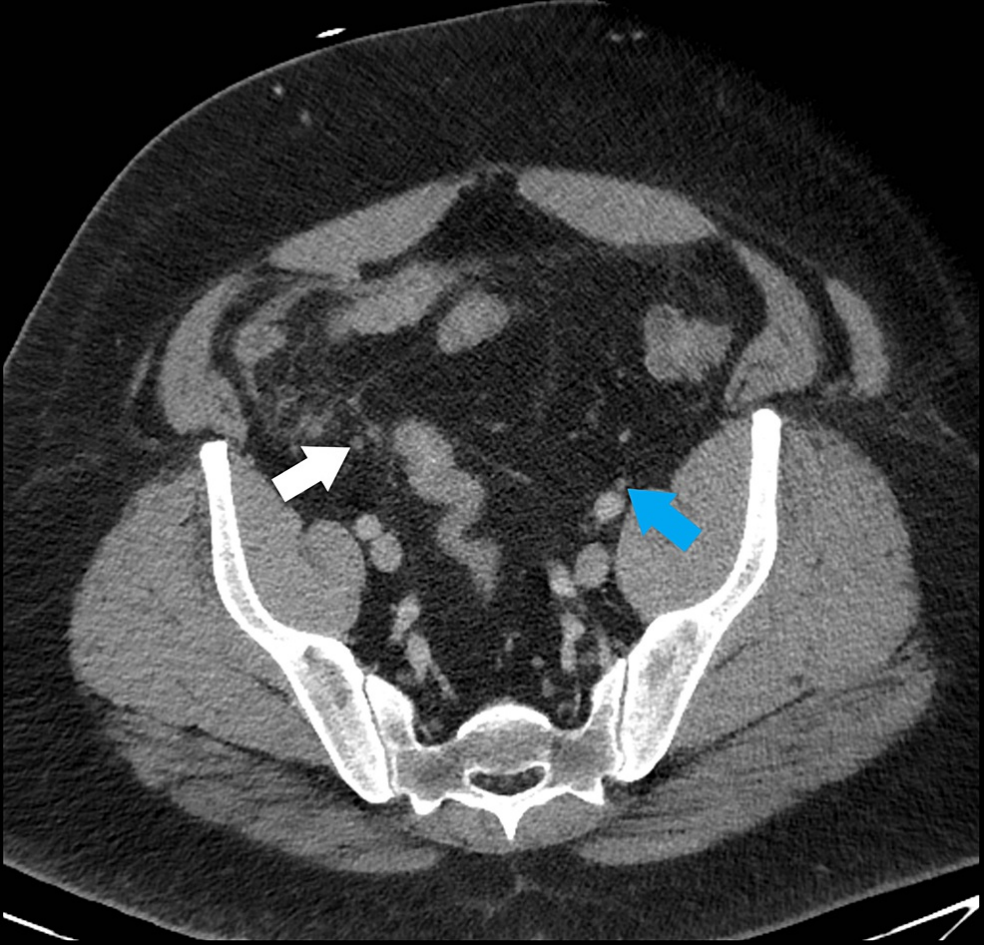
Pre-operative CT showing right ureter (white arrow) coursing far more anteriorly than left ureter (blue arrow).
